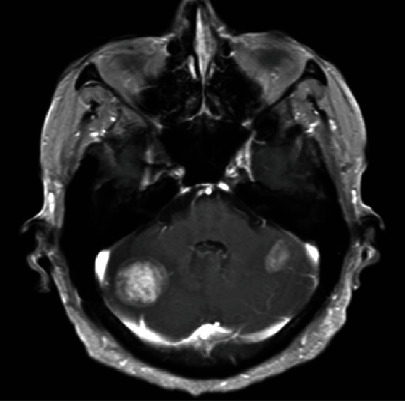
CT imaging of the head demonstrated brain metastasis.
